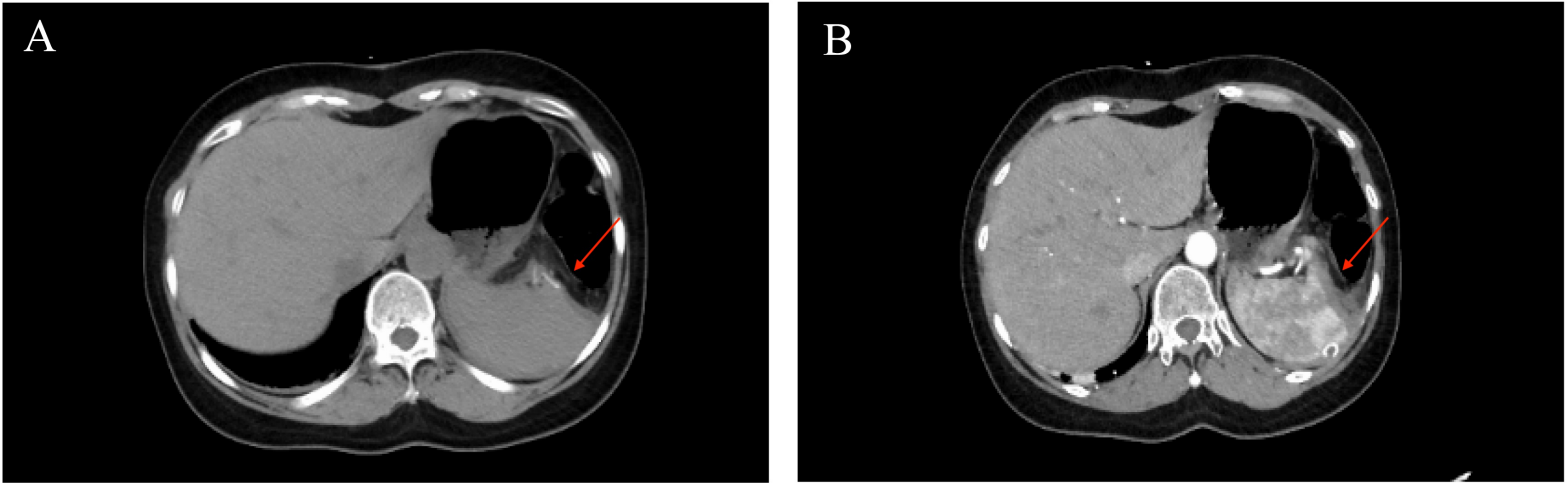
Postoperative computed tomography image. The red arrow (A, B) shows the spleen section, and no perisplenic blood or fluid accumulation was observed.
